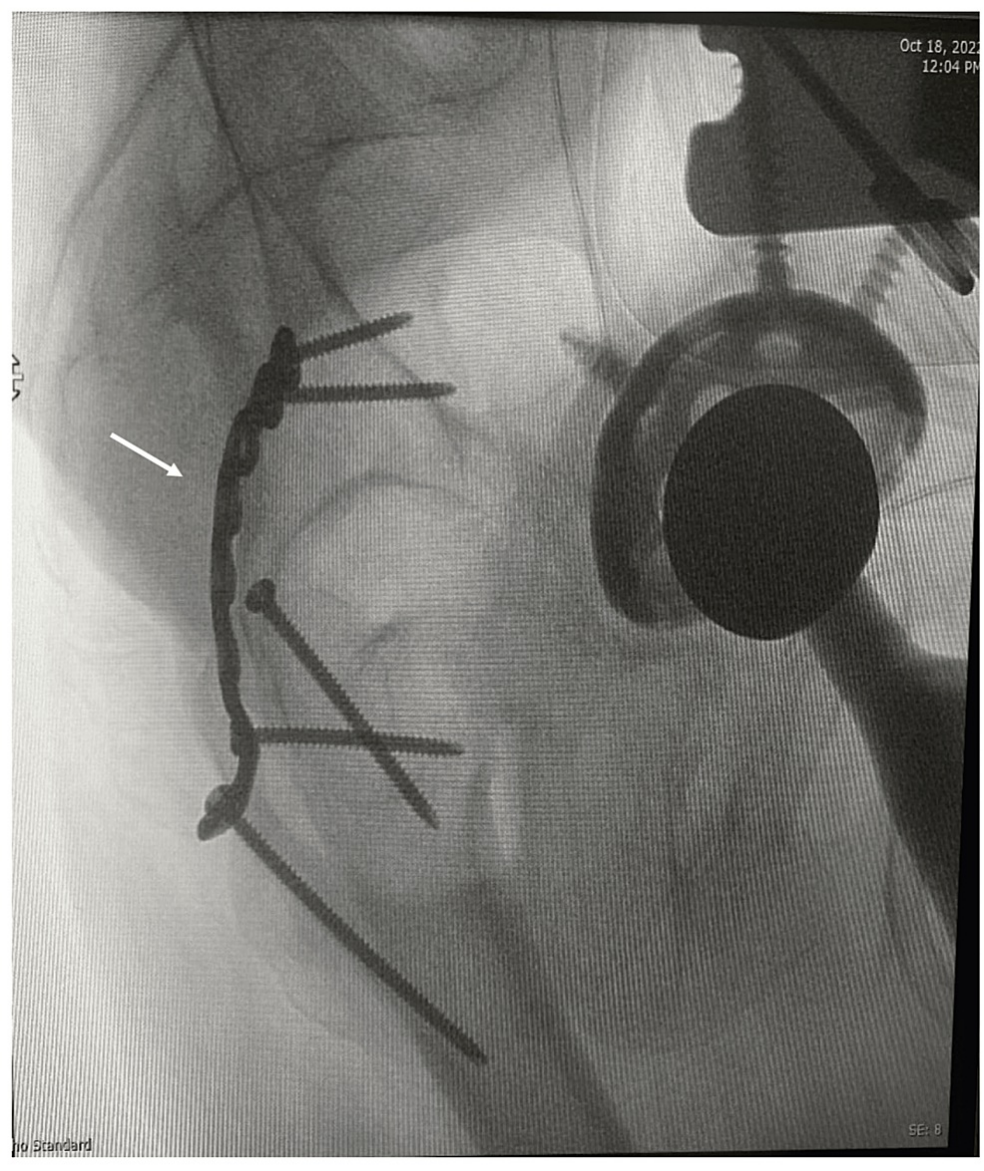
Intra-operative lateral pelvis x-ray showing fixation of the posterior column with plate and screws.
